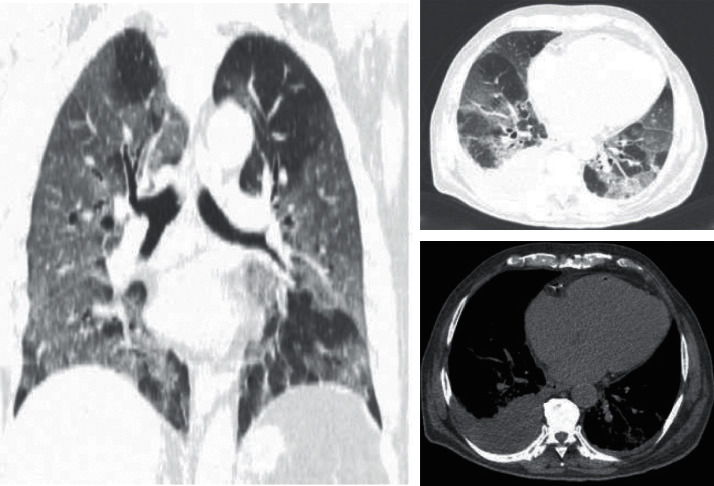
A 76-year-old male patient with diabetes deceased due to COVID-19. Chest CT scan ((a) coronal view, (b) axial view-lung window, and (c) axial view-mediastinal window) showed predominancy of ground-glass opacity (GGO) with the bilateral and diffuse distribution. Pulmonary involvement (PI) and PI density scores were 16 and 3.2, respectively. Additional findings included cardiomegaly and bilateral pleural effusion. He was considered a high-risk patient.
